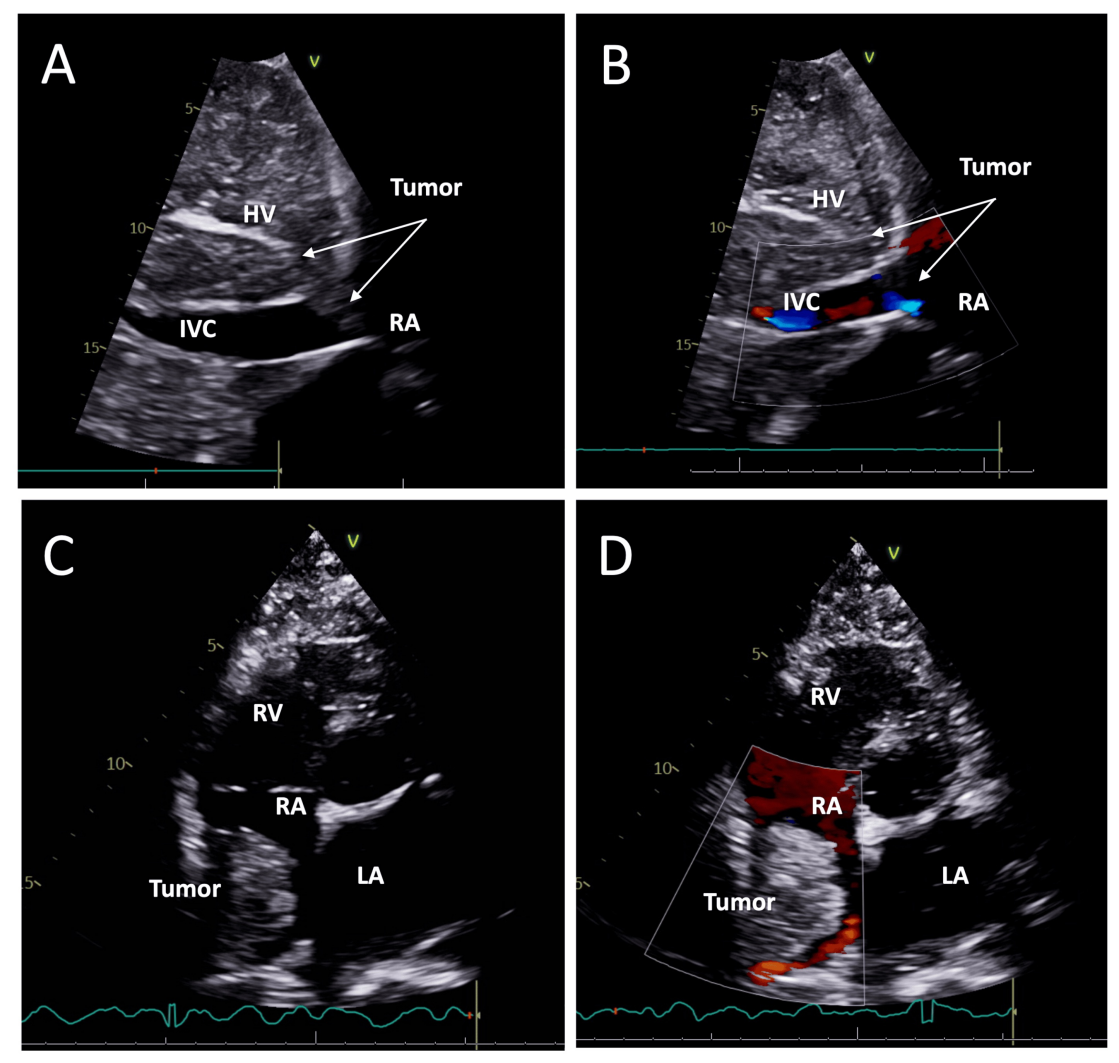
TTE revealed a large RA mass extending from the HV and IVC into the RA Subxiphoid view demonstrating a mass projecting from the HV into the IVC and RA on B-mode (A) and with color flow Doppler (B). Apical 4-chamber view showing a large RA mass on B-mode (C) and with color flow Doppler (D). TTE: transthoracic echocardiogram; IVC: inferior vena cava; RA: right atrium; RV: right ventricle; LA: left ventricle; HV: hepatic vein
